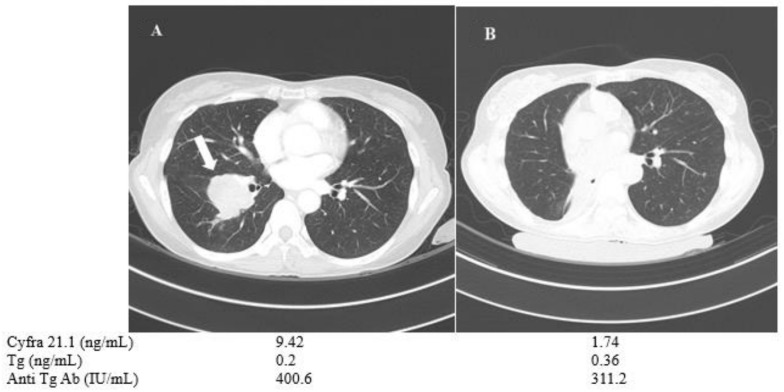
Change in chest CT images and serum biomarkers of a 50-year-old PTC female with Anti-Tg Ab (patient No. 8). The patient’s 4.6 cm tumor mass (arrow, A) was removed via bilobectomy of right middle and right low lobes (B).
